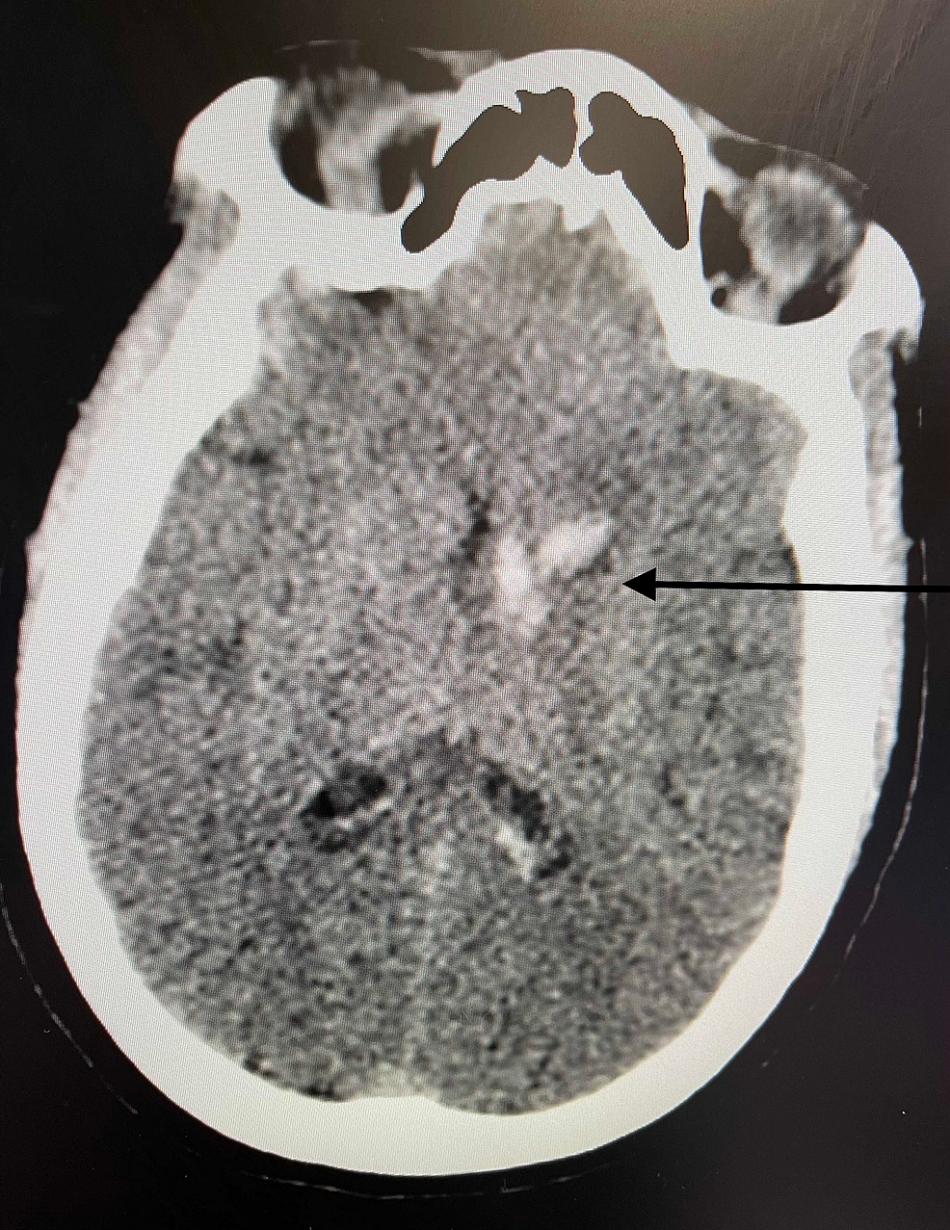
Initial head CT demonstrating left caudate nucleus intracerebral hemorrhage with left lateral ventricle intraventricular hemorrhage (arrow) CT: computed tomography
